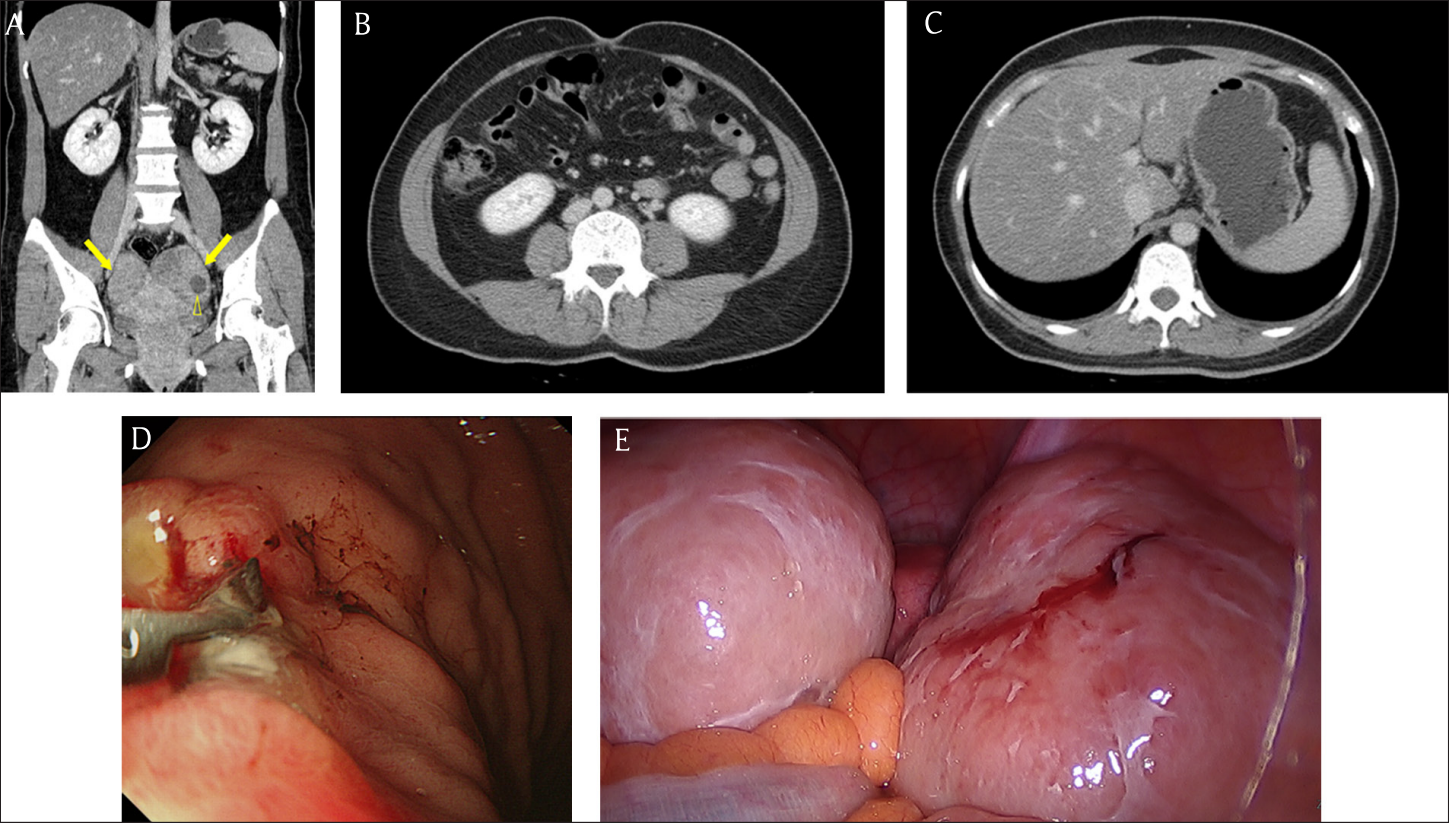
A 40-year-old woman with incidentally detected bilateral ovarian masses. (A) Coronal reformatted contrast-enhanced CT image demonstrated the heterogeneous enhancing masses (arrows) in the bilateral ovaries. Note the small cystic component (open arrowhead) within the ovarian mass. (B, C) There was no evidence of ascites, peritoneal thickening, abnormal lymph nodes, or a malignant tumor within an intra-abdominal solid organ or the gastrointestinal tract on the CT image. (D) Gastric adenocarcinoma with signet ring cell component was confirmed by endoscopy. (E) After surgical resection, the masses were diagnosed as Krukenberg tumors. Other peritoneal seeding lesions were detected in the surgical field.
